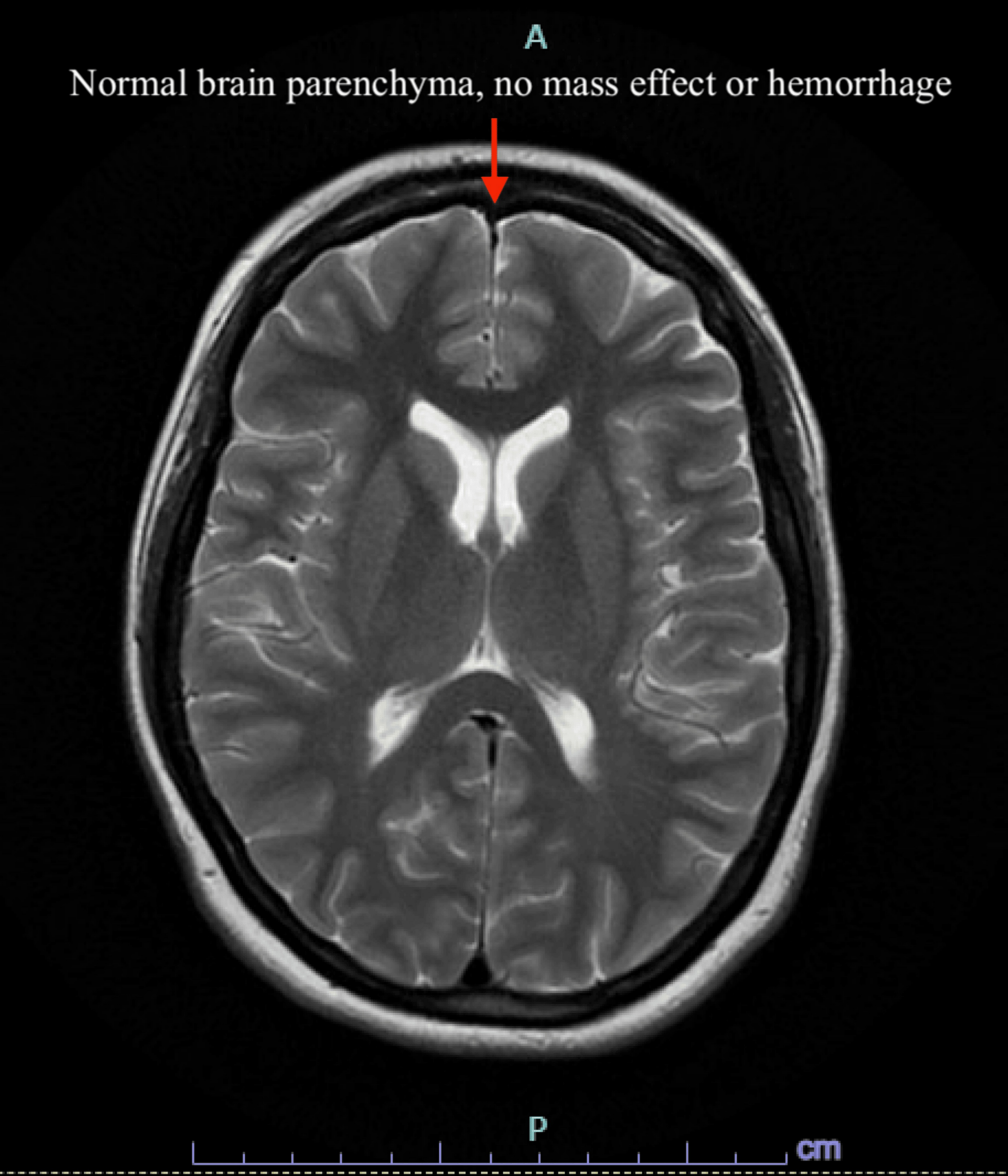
MRI of the Brain Demonstrating No Acute Abnormalities
